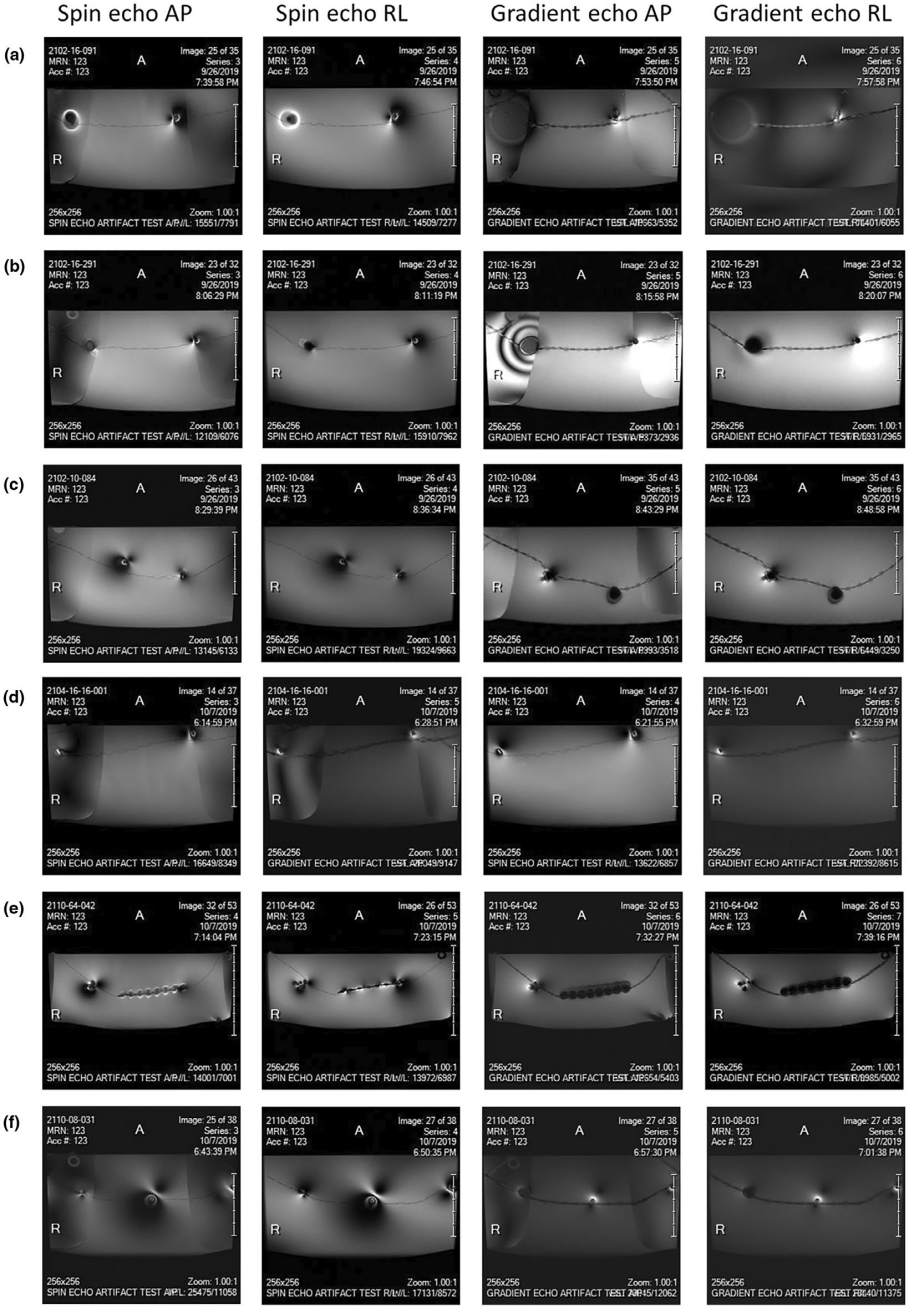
Images of the expected distortion and signal loss artefacts produced in MR imaging of test articles. (a) 16‐contact standard depth electrode ID# 2101‐16‐091, (b) 16‐contact RF ablation depth electrode ID# 2102‐16‐291, (c) 10‐contact depthalon depth electrode ID# 2102‐10‐084), (d) 8‐contact depth + 16‐contact microwire electrode ID# 2104‐16‐16‐001, (e) 64‐contact Contac grid ID# 2110‐64‐042, and (f) 8‐contact Contac strip ID# 2110‐08‐031.
